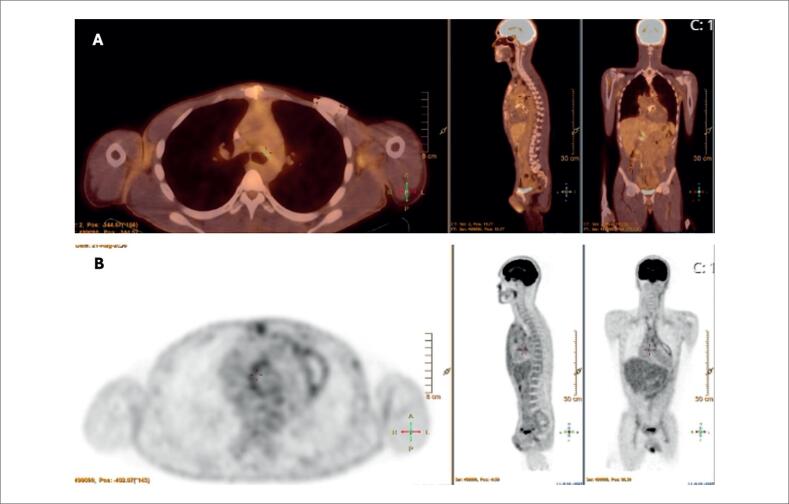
PET/CT com ^18^F-FDG pós-operatório do paciente. A - Ausência de hipermetabolismo focal anormal, confirmando a ausência de infecção residual da prótese valvular. B - Captação perivalvar difusa compatível com alterações pós-operatórias recentes.
